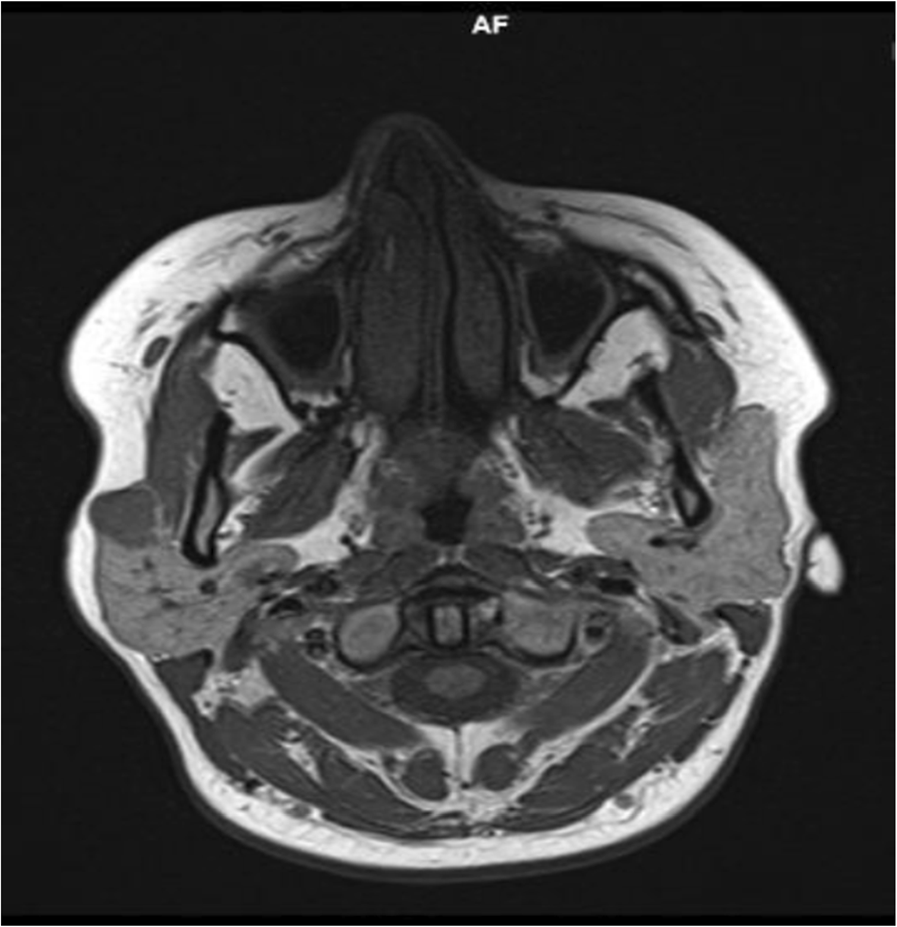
Axial T1-weighted image with hypointensity showing the tumor process of the right parotid gland
